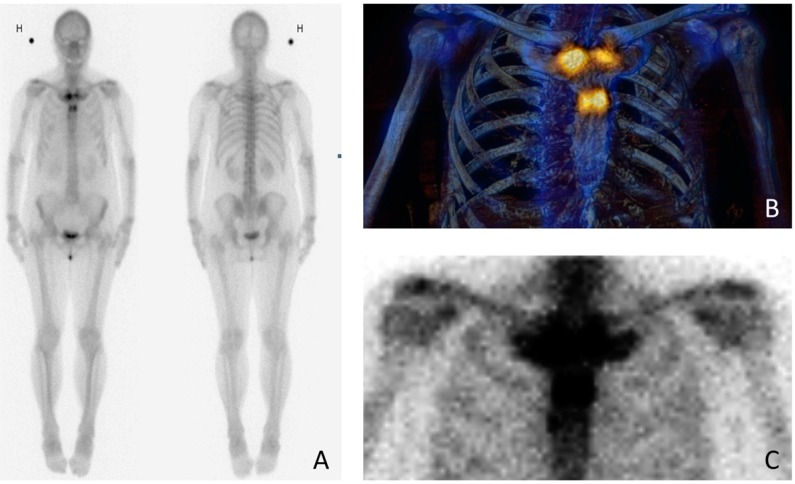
To rule out malignancy, the patient was examined with whole-body bone scintigraphy with both planar (A) and single photon emission computed tomography (SPECT) (B)/low-dose computed tomography (CT) images (C). Scintigraphy was performed in 2015, within two weeks of the chest radiograph (Figure 1A). The images demonstrate abnormal radiotracer activity in both sternocostoclavicular joints, in the sternal angle and manubrium and also in the proximal body of the sternum. The symmetric tracer activity adjacent to the joints rules out malignancy; instead, scintigraphy shows a typical “bullhead sign” [7]. The bullhead sign consists of increased scintigraphic activity in the sternal manubrium and in the adjacent clavicles and ribs, representing the skull and horns of the bull, respectively. The increased scintigraphic activity corresponds to the radiographic changes caused by the hyperostosis. The bullhead sign is a characteristic scintigraphic pattern in demonstrating SCCH [7,8,9]. H stands for højre (Danish) = right side of the patient. The possibilities of diagnostic imaging are wide. Chest radiographs, Tc-99 m bone scintigraphy, computed tomography (CT) or magnetic resonance imaging (MRI) are all used to various extent [4,5,7,10]. Whole-body bone scintigraphy and MRI (including T1-weighted (T1W), short tau inversion recovery (STIR) with or without T1W post-gadolinium sequences) have both proven to be of great importance where radiographically occult sites hamper the diagnosis [5,7]. In case of extensive follow-up imaging, MRI has the advantage of being radiation-free.
